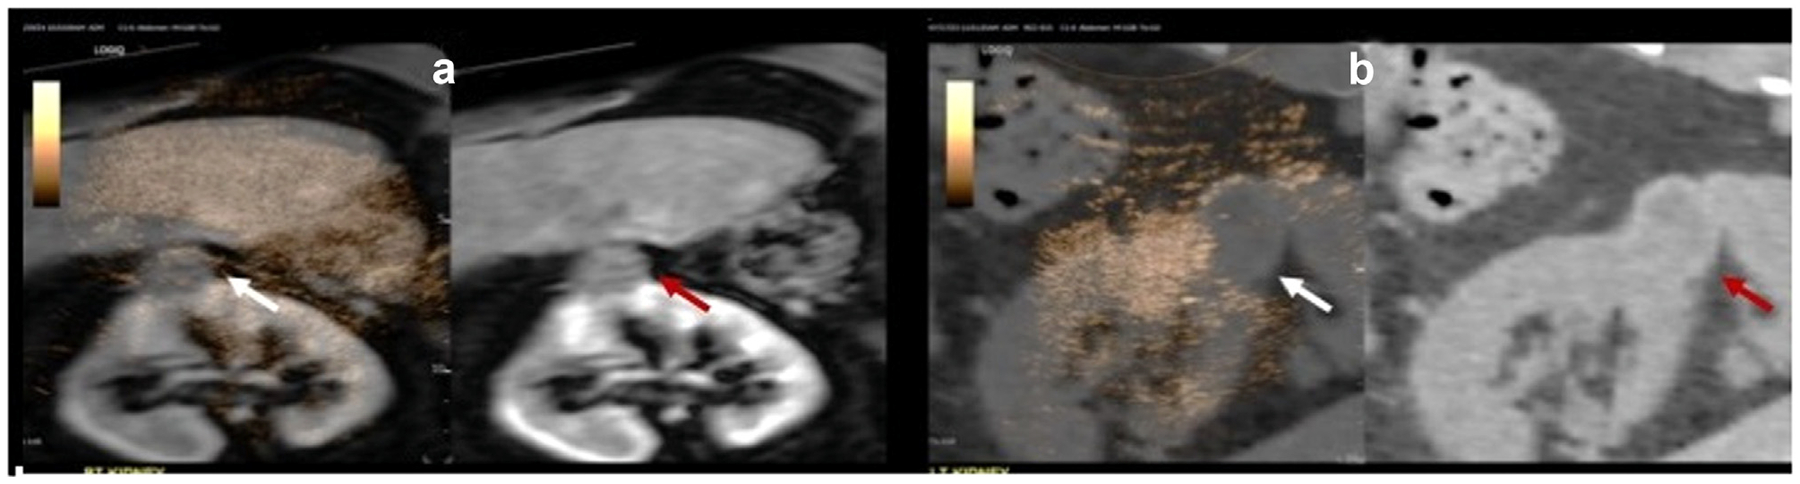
(a) An example of an excellent overlay of patient’s CEUS and preablation MRI from 4 years prior. (b) An example of a challenging fusion of patient’s CEUS and preablation CT three years prior.
